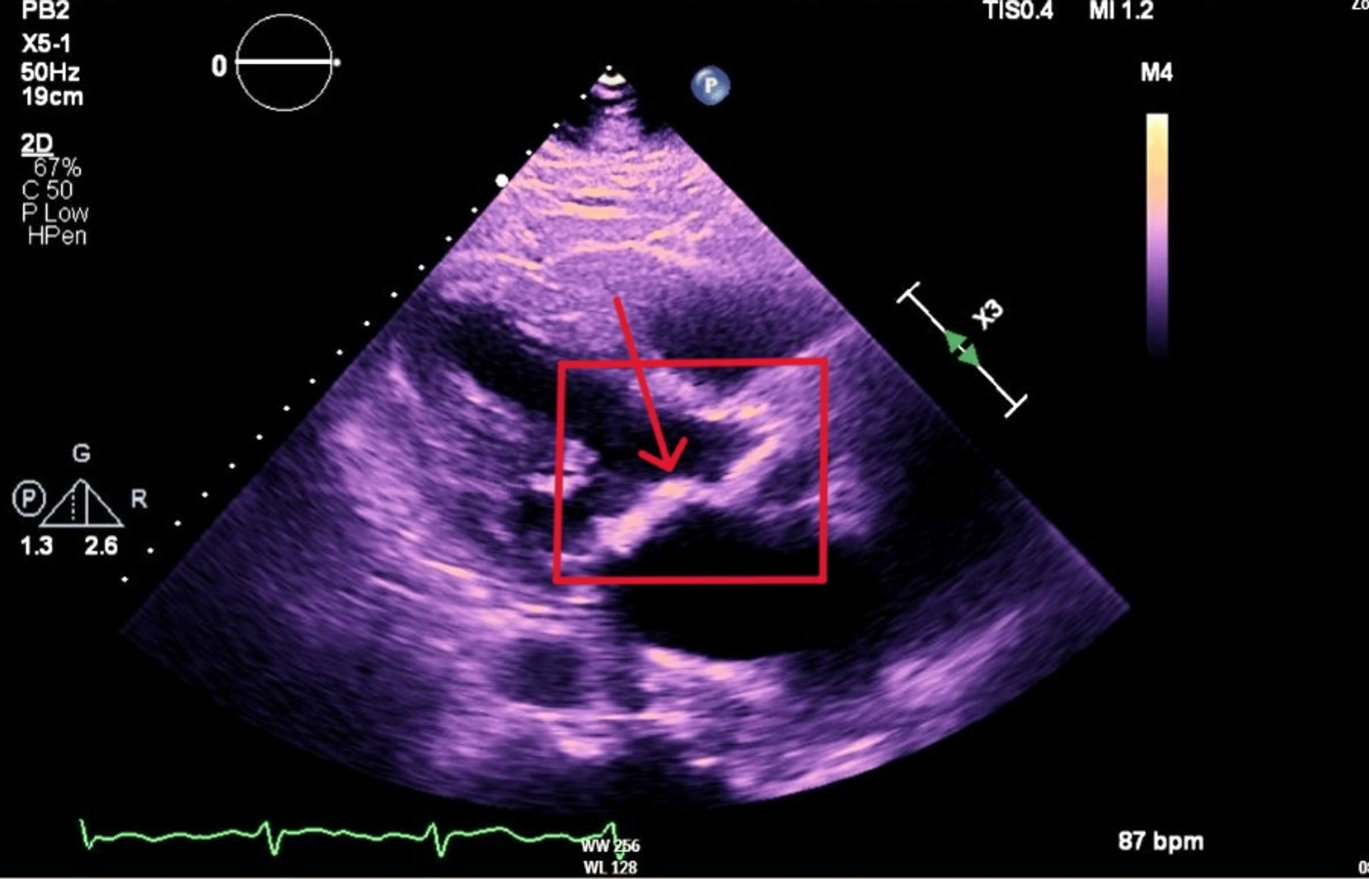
Parasternal view of mitral valve stenosis on echocardiogram (red arrow in red box)
